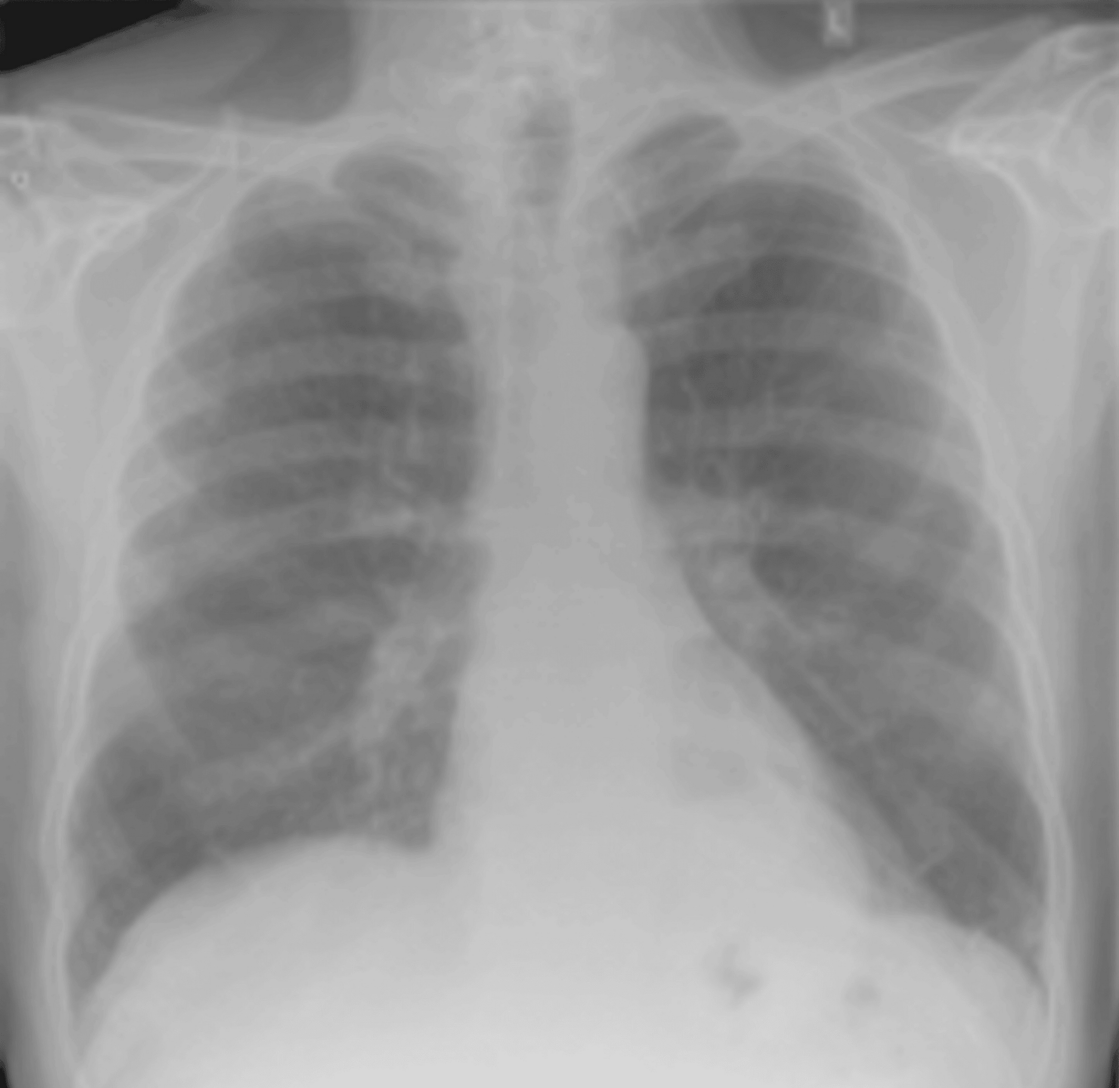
Chest X-ray with normal findings
